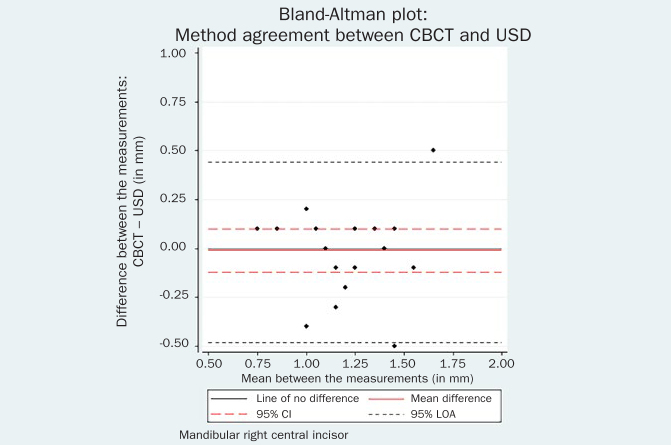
Bland-Altman plot CBCT vs USD 41.
